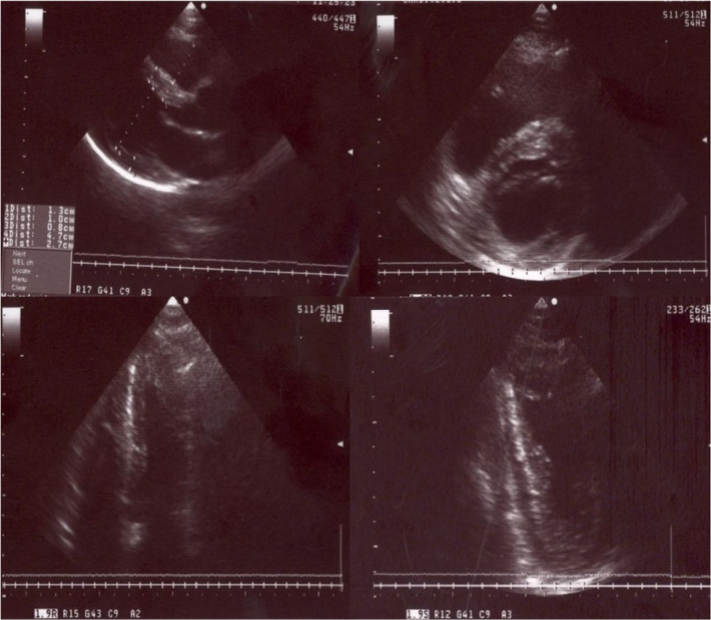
Baseline echocardiography showing normal cardiac cavities, septal hypokinesia, left ventricular apical akinesia and ballooning.
